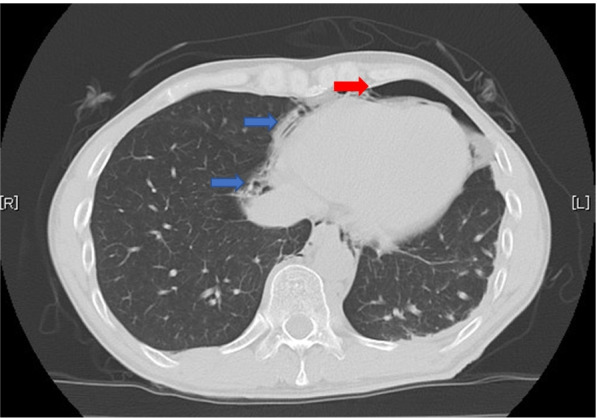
Computed tomographic images. Thoracic computed tomography showing a left-sided pneumothorax (red arrow) and mediastinal emphysema (blue arrow)
